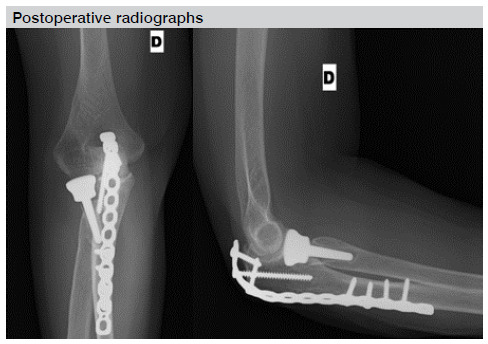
Osteolysis around the radial head prosthesis as a complication. Postoperative radiographs
